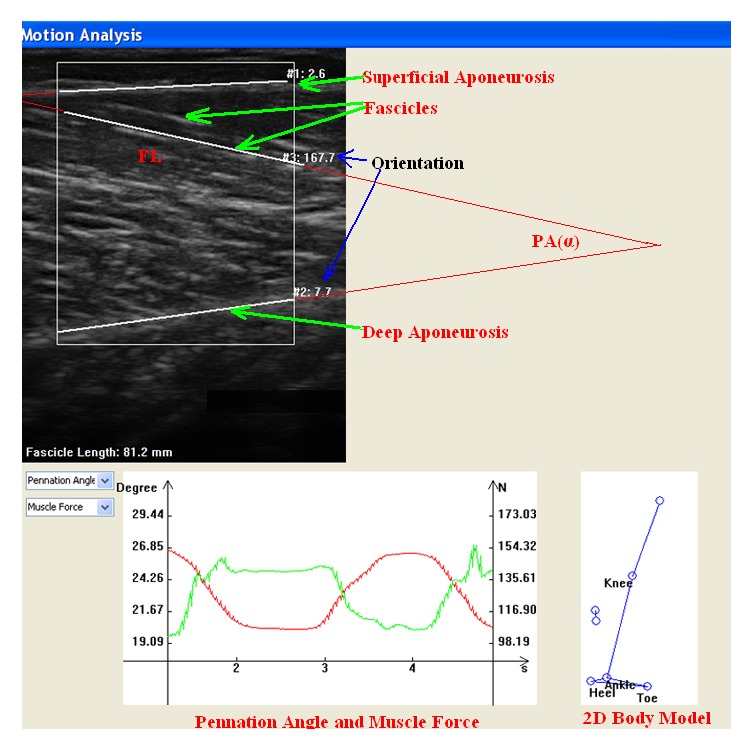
The FL and PA as defined in GM ultrasound image on the interface of custom-designed data analysis platform.
